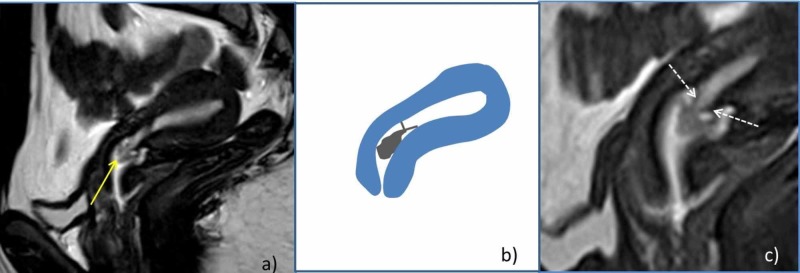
Pelvic magnetic resonance images T2-weighted sagittal images (a, c), the yellow arrow shows an endometrial heterogeneous lesion adjacent to the internal cervical os, which corresponds to an endometrial polyp. The white arrows in Figure 2c signal two thin band-like hypointense structures that partially obstruct the endometrial cavity. Figure 2b shows a representation of the endometrium-dependent structure and its anatomical location.
